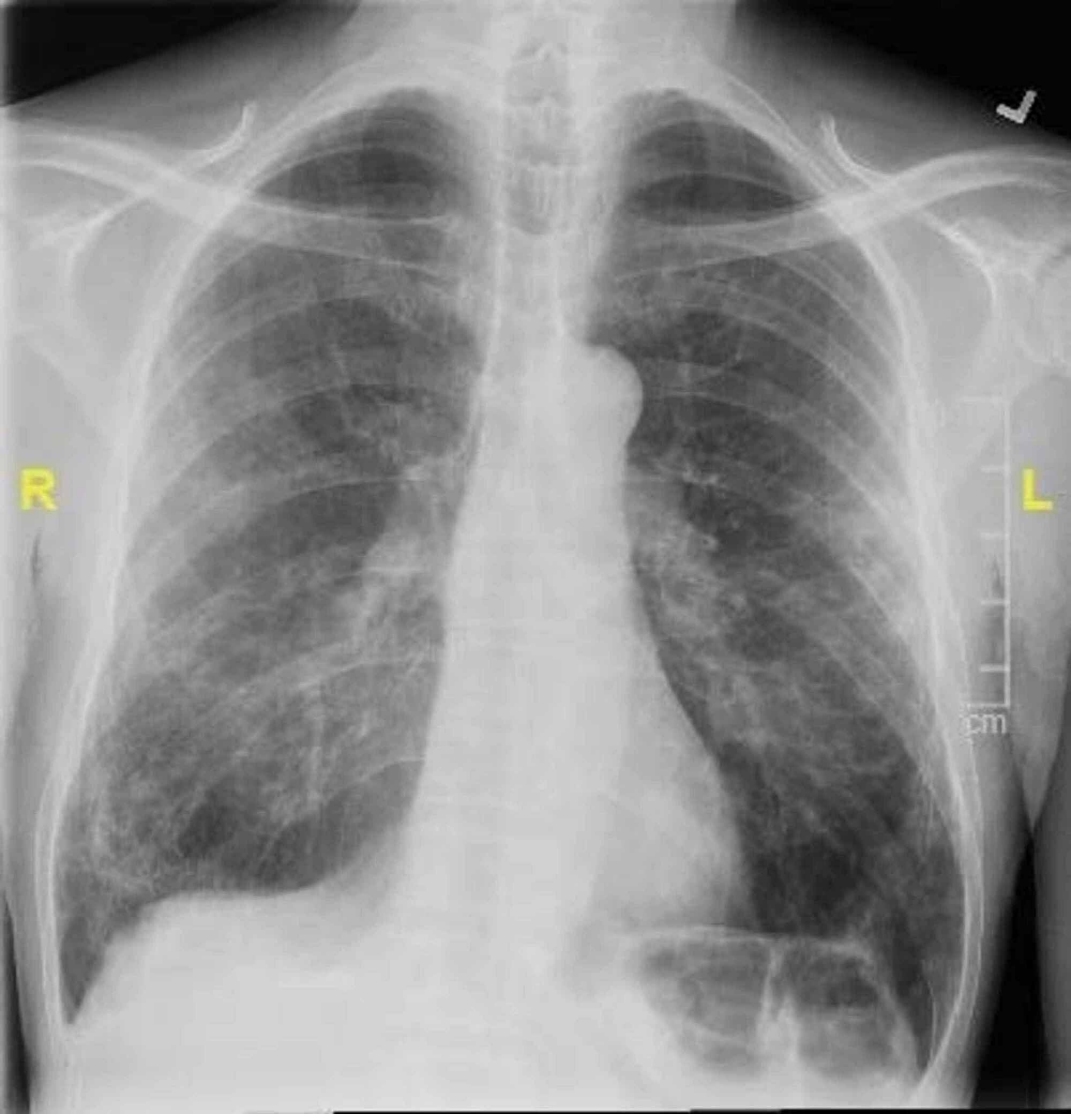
X-ray chest demonstrates bilateral opacities
